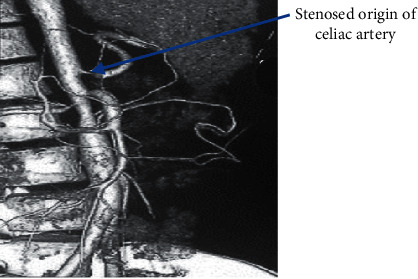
CTA showing the stenosed origin of the celiac artery.
